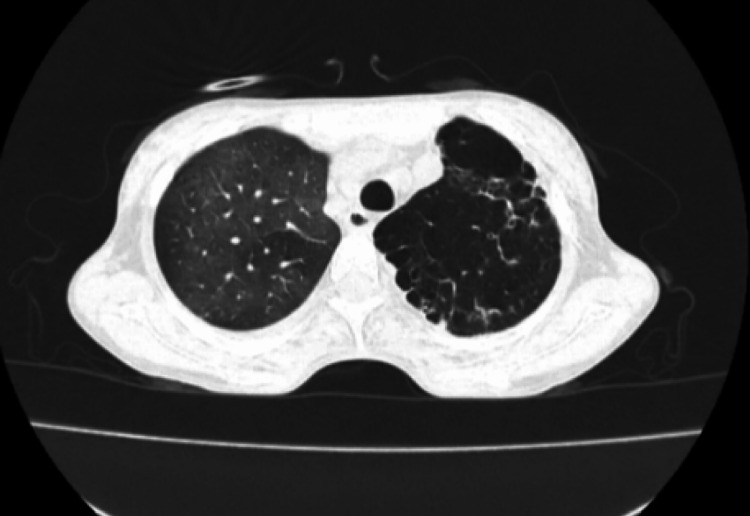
Computed tomography of the thorax
